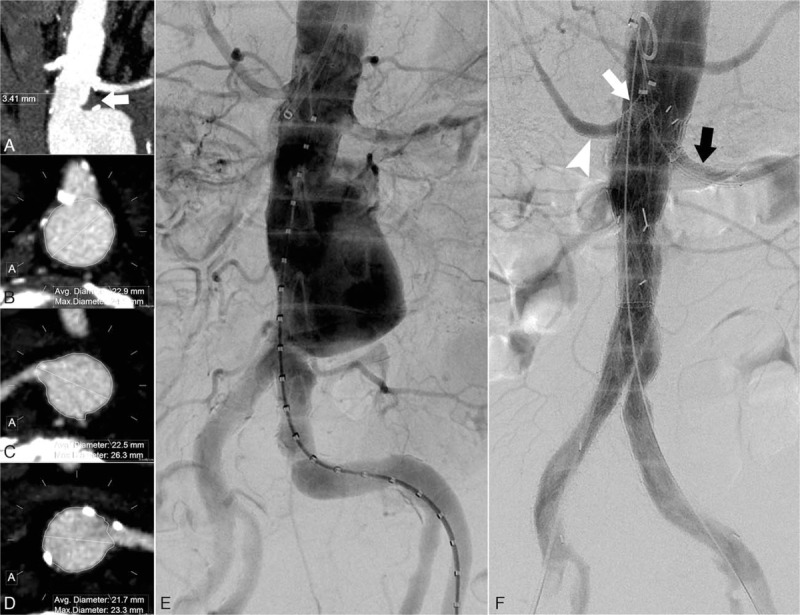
The preoperative CTA. (A) Imminent rupture of the abdominal aortic aneurysm, with an aneurismal neck length of 3.4 mm and exudation near the aneurismal neck (white arrow in A). Preoperative measurement of the positions of the superior mesenteric artery (B) and bilateral renal arteries (C, D) was conducted by means of clock-face orientation. Preoperative digital subtraction angiography (DSA) (E) revealed an abdominal aortic aneurysm with hostile neck anatomy. Endovascular repair of the abdominal aortic aneurysms was carried out with the reconstruction of the mesenteric artery using a balloon-expandable stent (Scuba, InvatecS.pA, Italy) (white arrow in F). After the reconstruction of the right renal artery using a balloon-expandable stent (Paramount Mini GPS; ev3 Inc.) (white arrowhead in Fin F), a dissection of the left renal artery was found. The stent graft (Viabahn; WL Gore & Associates, Inc., Flagstaff, AZ) and self-expanding stent (Zilver, Cook Ireland Ltd., Limerick, Ireland) were used to extend into the left renal artery (black arrow in F). The postoperative DSA (F) showed that the blood flow of bilateral renal arteries was smooth. CTA = computed tomographic angiography, DSA = digital subtraction angiography.
